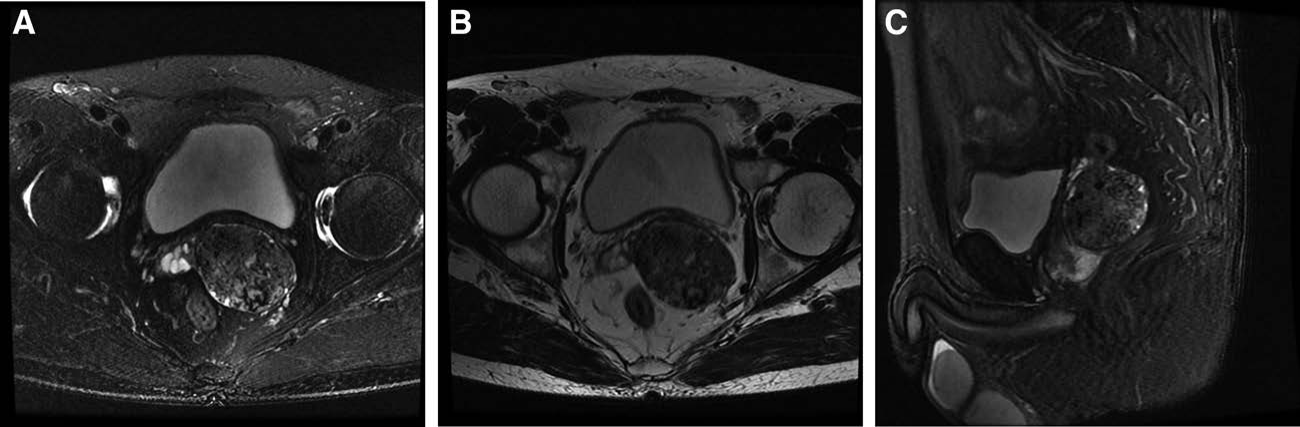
(A–C) Prostate magnetic resonance imaging (MRI) shows an increase in prostate volume, with T1 and T2 mass signals protruding to the left and rear in the left central zone of the prostate, and spotted high signal in DWI. DWI = diffusion weighted imaging.
